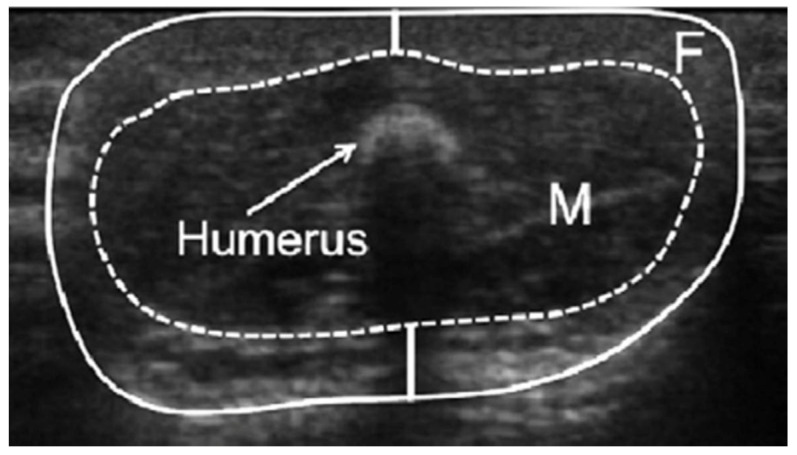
Ultrasound measurements of fat and lean areas of the infant’s arm. F, fat area; M, lean area (muscle and bone).
